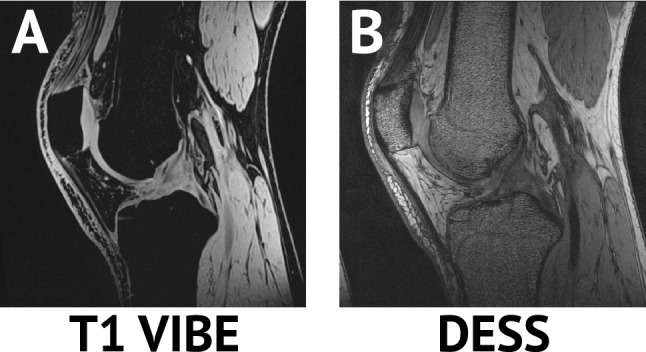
Sagittal (A) T1 VIBE and (B) DESS MR images were acquired of each human participant’s dominant knee. While DESS images have been reliably used for knee joint bone and cartilage model generation in the past, T1 VIBE had greater contrast between the bone and adjacent cartilage (0.95 vs. 0.72). *DESS* Double Echo Steady-State, *T1 VIBE* T1-weighted Volume-Interpolated Breathhold Examination with Water Excitation.
